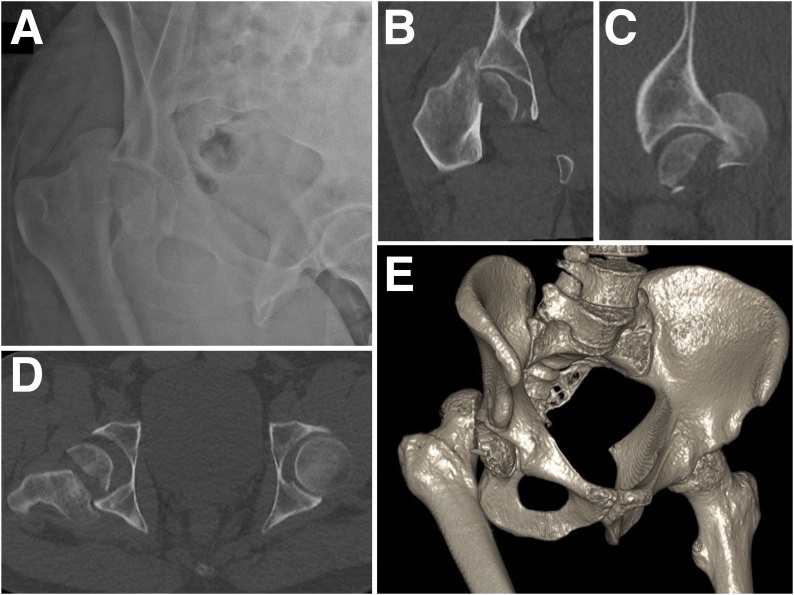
The initial anteroposterior (AP) pelvic X-ray (1A) and Pelvic CT images showing posterior dislocation and Pipkin II fracture of the femoral head, with a large fragment remaining in the acetabulum (1B: frontal view; 1C: sagittal view; 1D: axial view; 1E: three-dimensional reconstruction).
